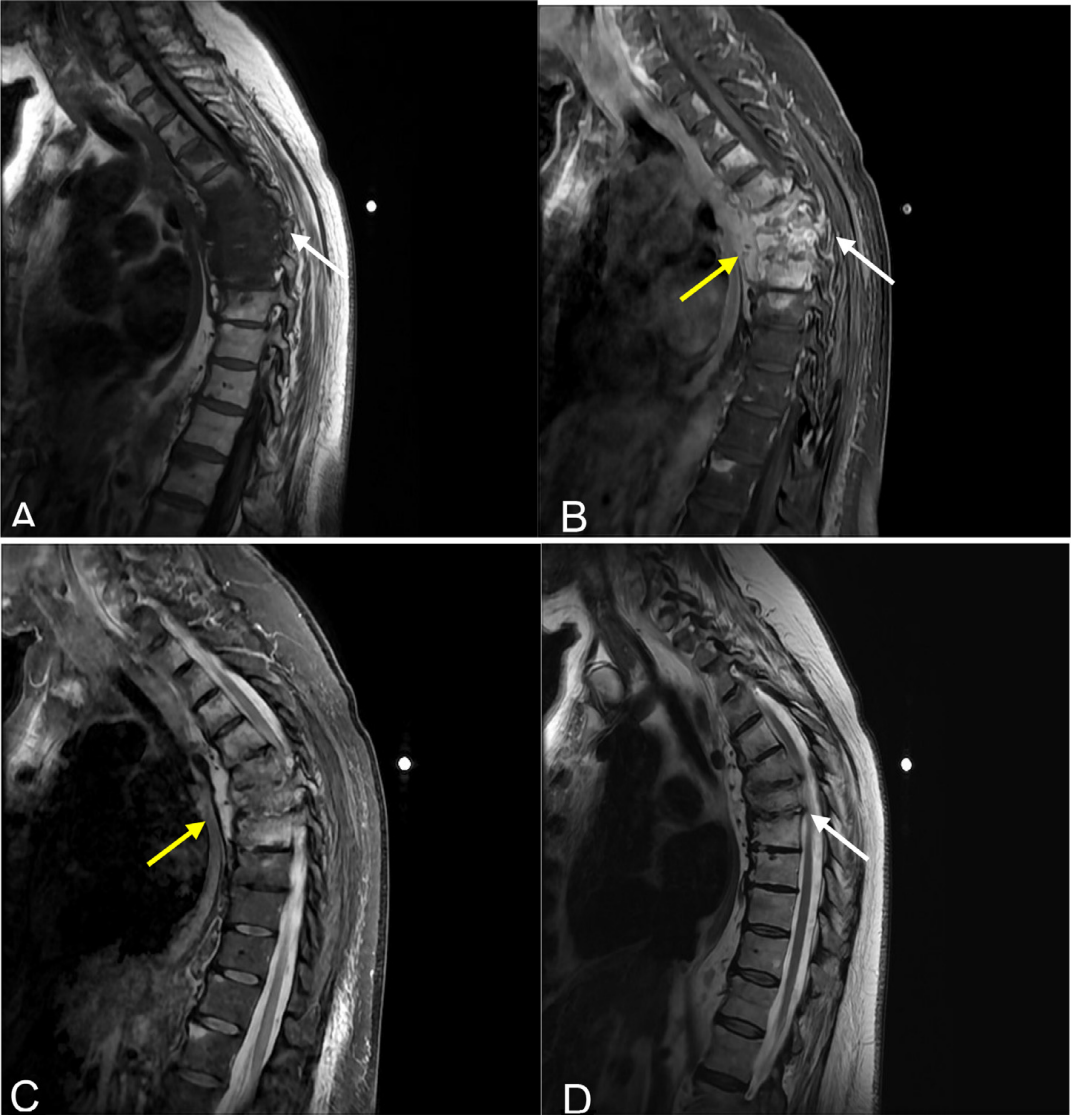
MRI thoracic spine sagittal: (A) T1 fluid attenuated inversion recovery (FLAIR), (B) postcontrast T1 with fat suppression, (C) STIR, and (D) T2. (A&B) Presence of extensive enhancing bone marrow changes at T5-T9 (white arrows) with (B&C) associated paravertebral phlegmon enhancement (yellow arrows). (D) Anterior wedge deformities of T6-T8 (white arrow). Additional skip lesions were noted in the cervical and lumbar spine (cervical involvement shown in Fig. 3).
